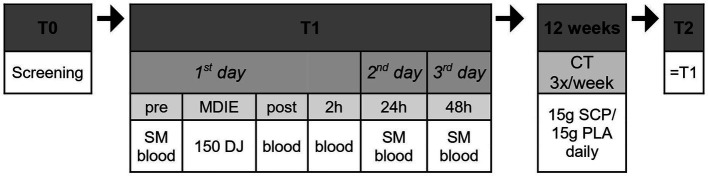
Study design in chronological order. Blood, blood collection; MDIE, muscle damage inducing exercise; DJ, drop jump; CT, concurrent training; SM, standardized meal.
